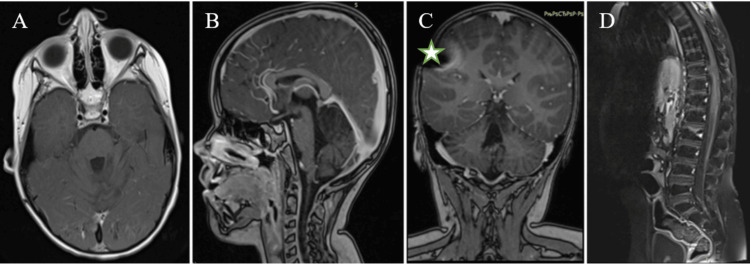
T1 contrast-enhanced MRI of the CNS four years after diagnosis No visible tumor lesions in the CNS. In the coronal section (C), a hypointense artifact is seen resulting from the VPS valve (white asterisk). CNS, central nervous system; VPS, ventriculoperitoneal shunt
